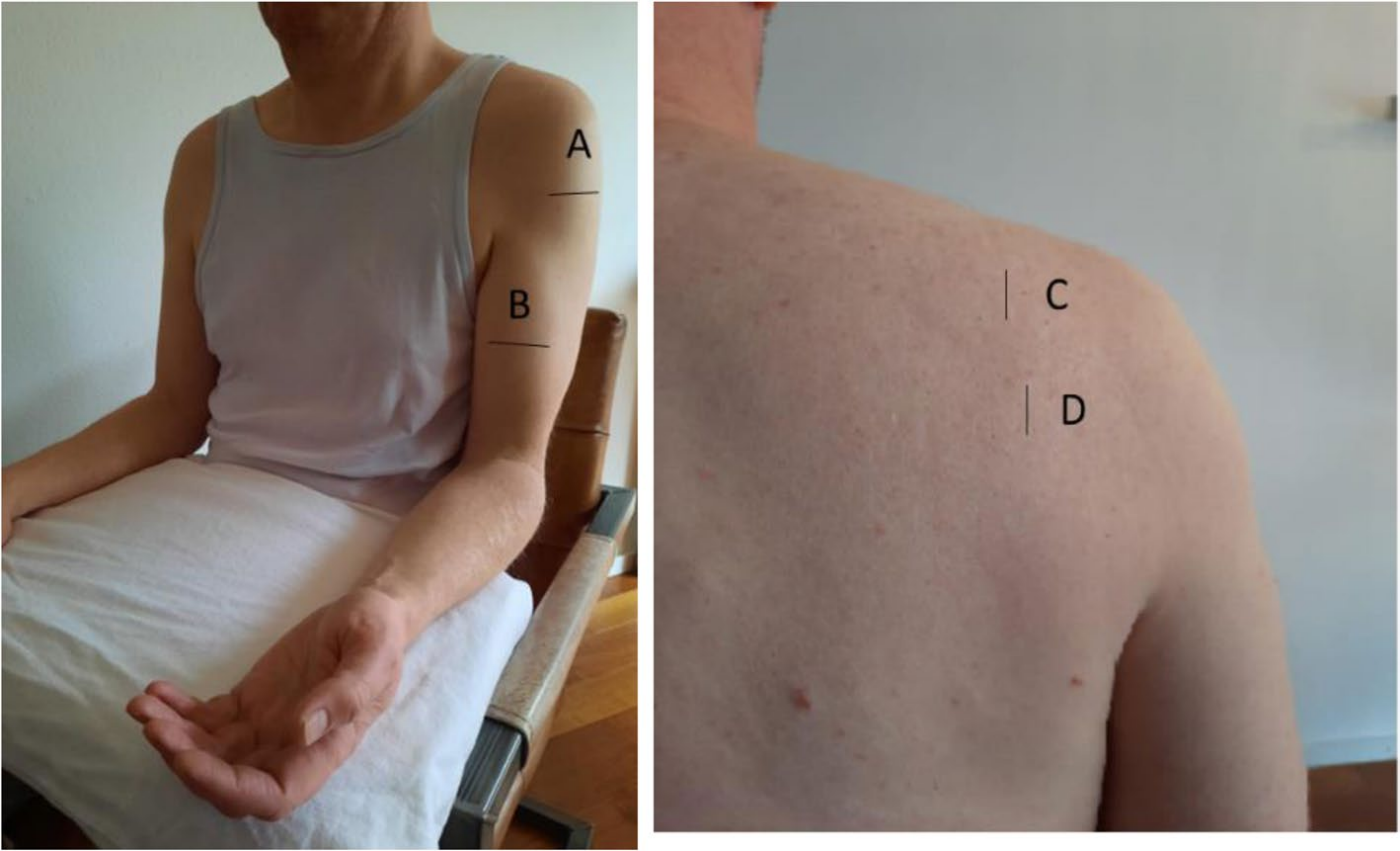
Scanning locations of the four investigated muscles for quantitative muscle ultrasound. A, location for deltoid muscle; B, location for biceps brachii; C, location for supraspinatus muscle; D, location for infraspinatus muscle
